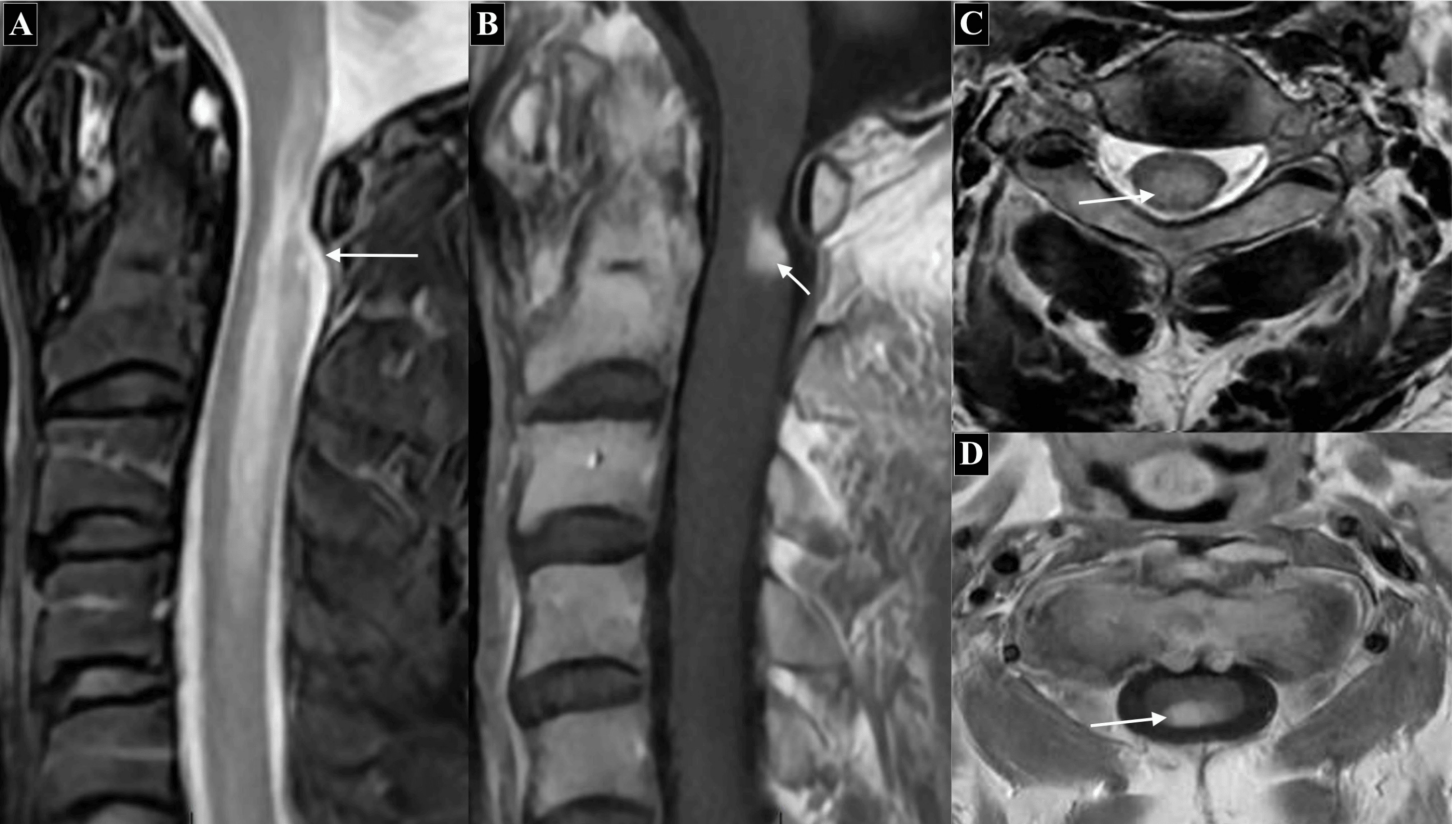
Preoperative T2 (A, C) and gadolinium-enhanced T1 (B, D) weighted axial MRI showed cervical T2 hyperintense lesion with focal nodular enhancement MRI: magnetic resonance imaging
